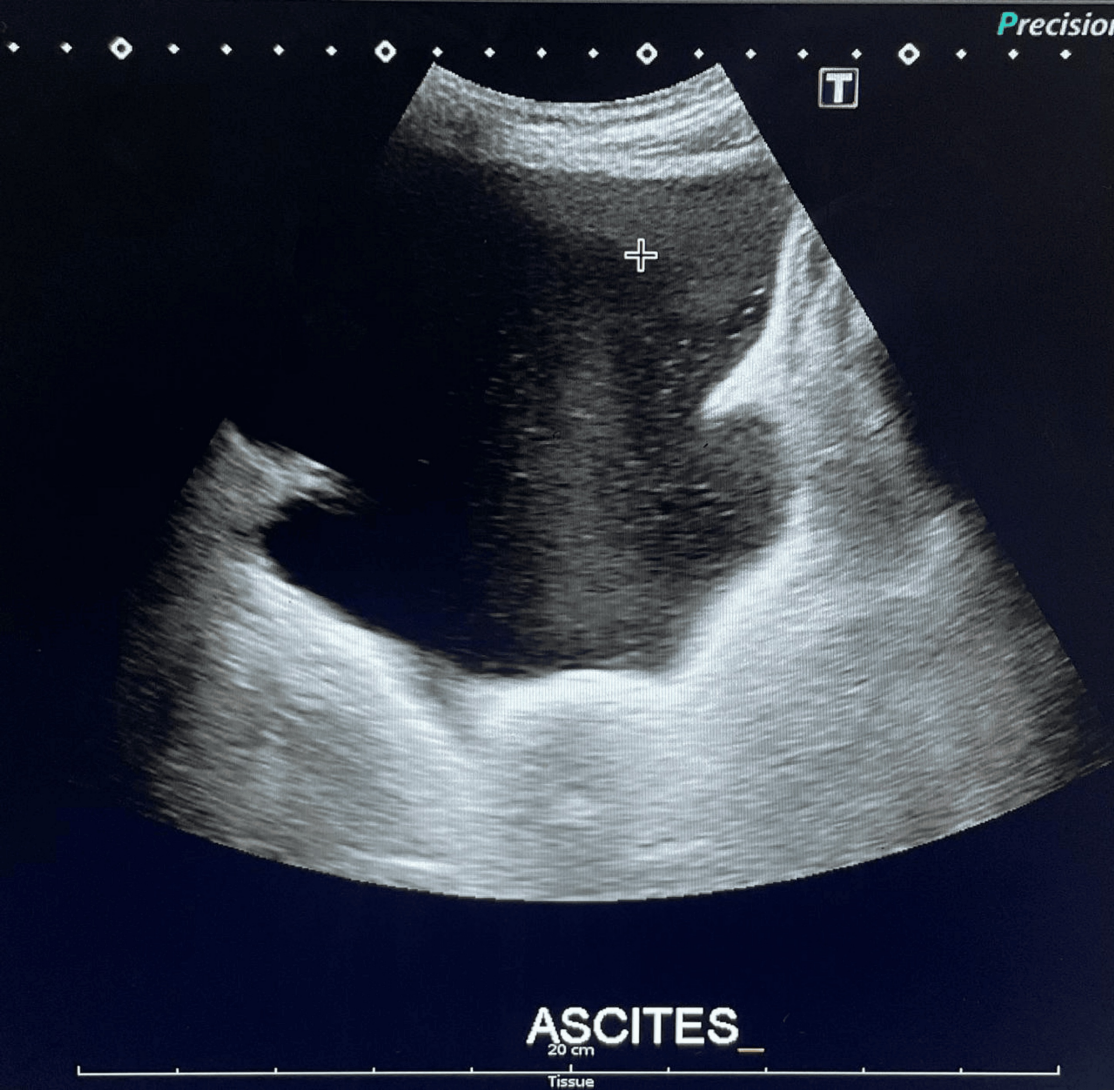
Ultrasound of pelvis revealing gross pelvic ascites with no adnexal masses
